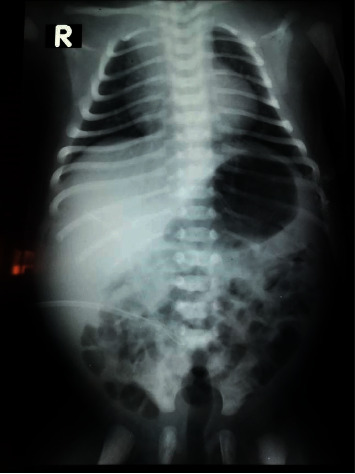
X-ray spine anteroposterior view: absent L5 vertebra and sacrum and bilateral iliac bones attaching to L4 vertebra.
